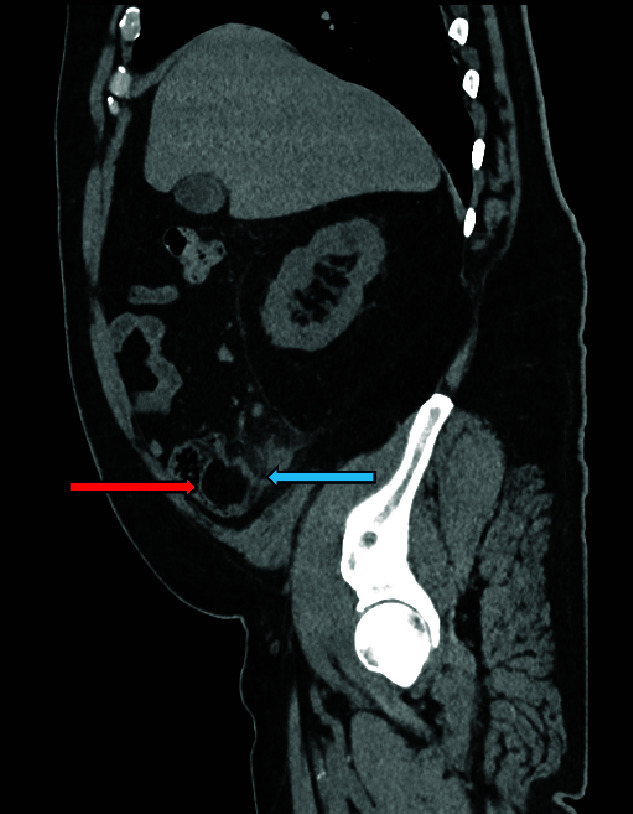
Sagittal CT image, showing caecal lipoma (right arrow) and appendicitis (left arrow).
